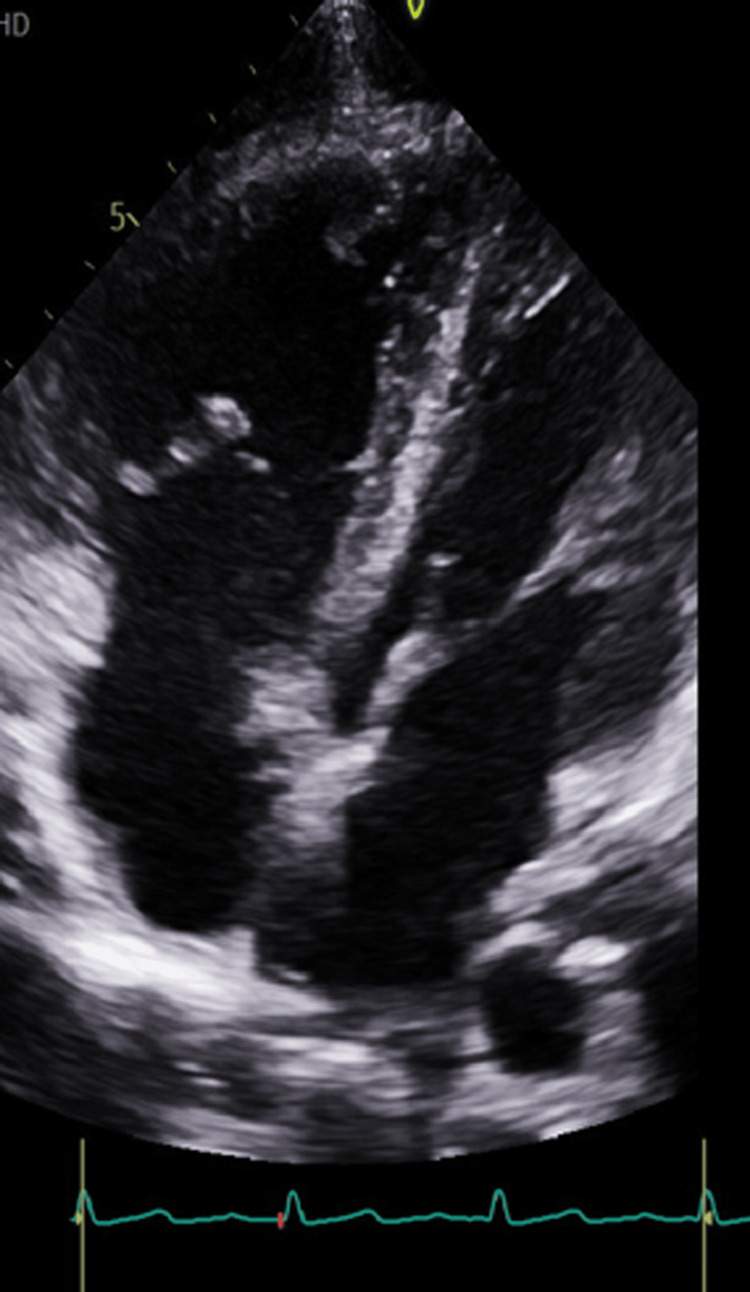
Transthoracic echocardiogram demonstrating flail anterior tricuspid leaflet with avulsed papillary muscle
